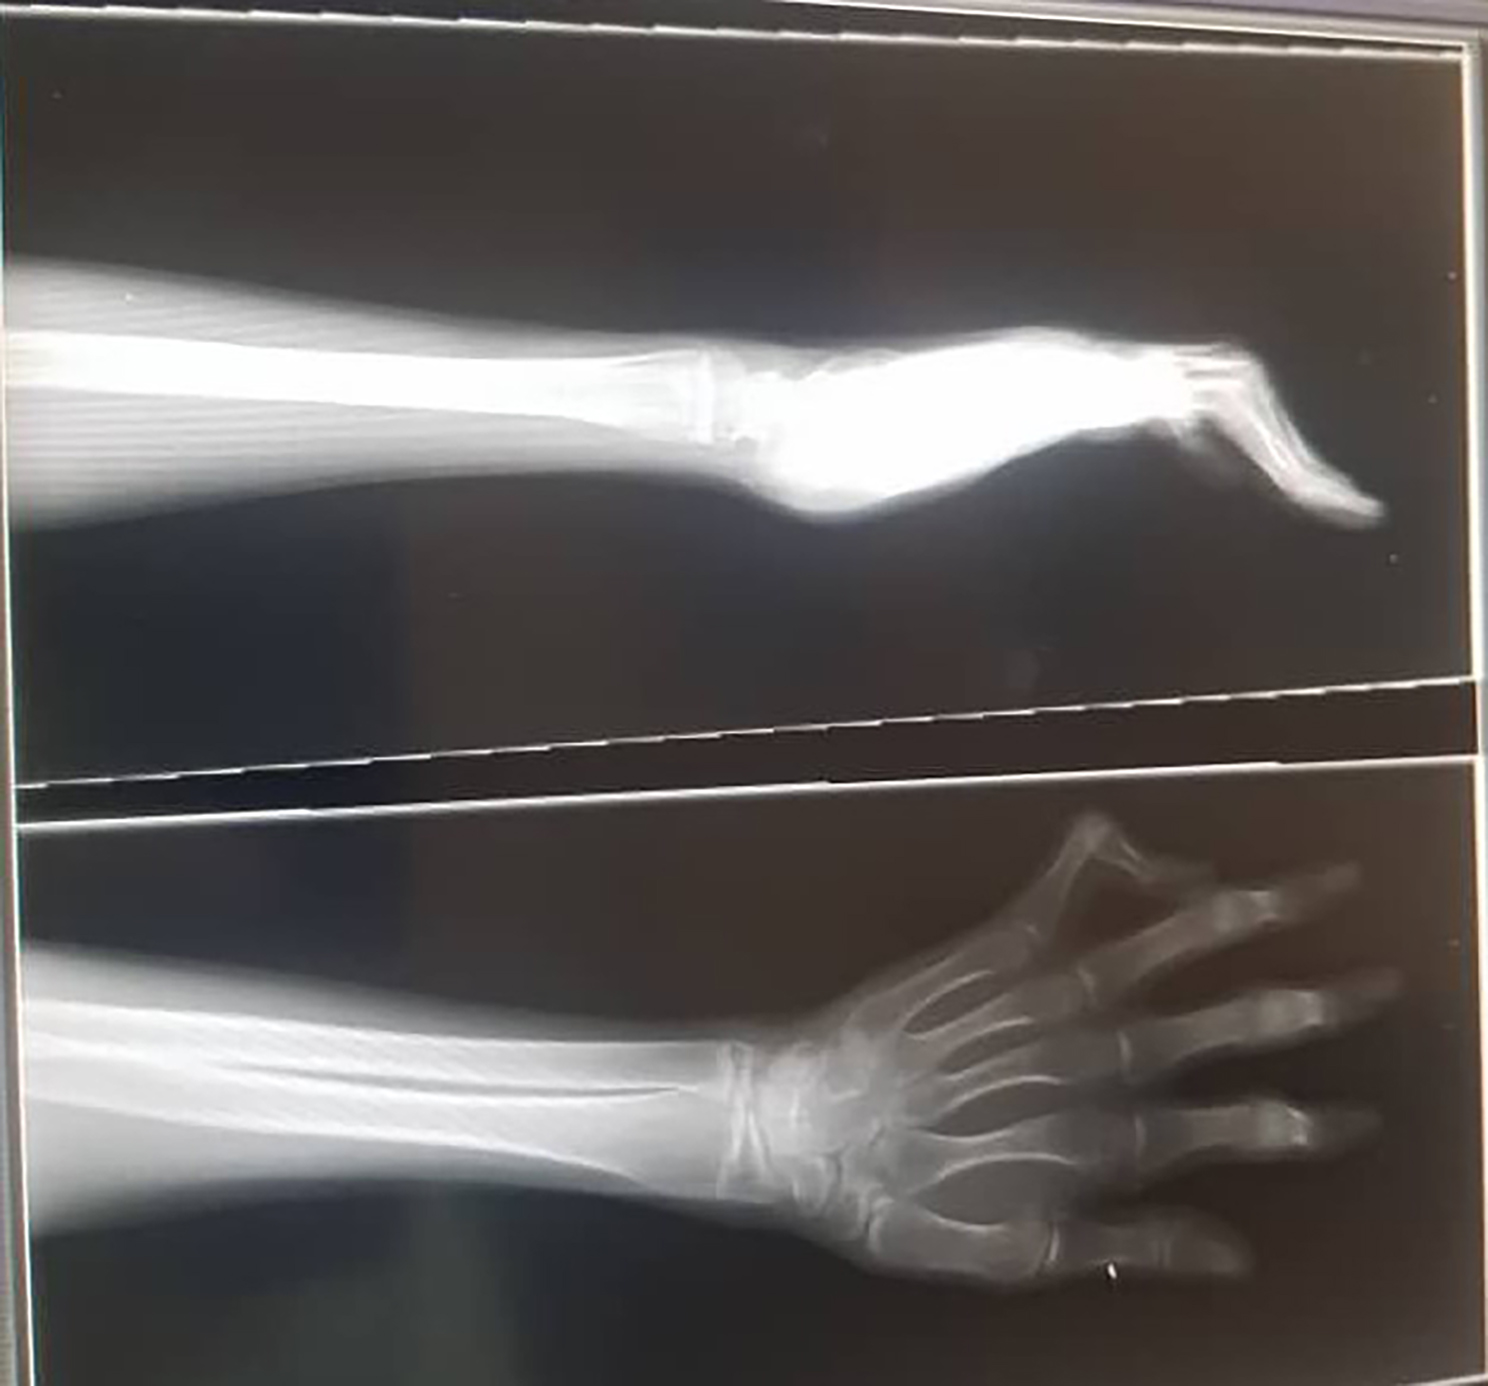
X-ray of the hand demonstrated Boutonniere deformity
